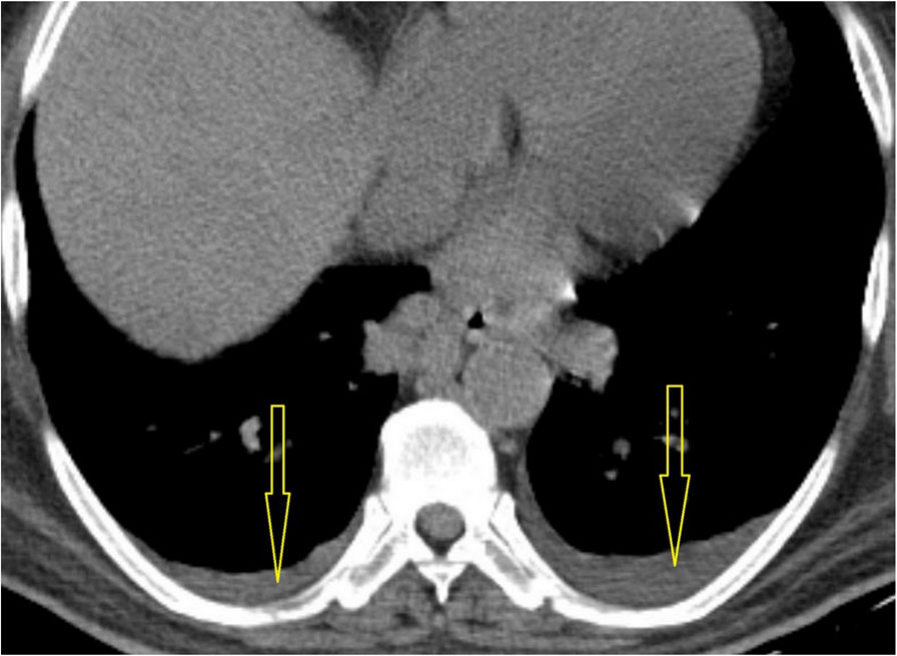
CT scan of COVID-19 patient shows minimal bilateral pleural effusion (yellow arrows)
